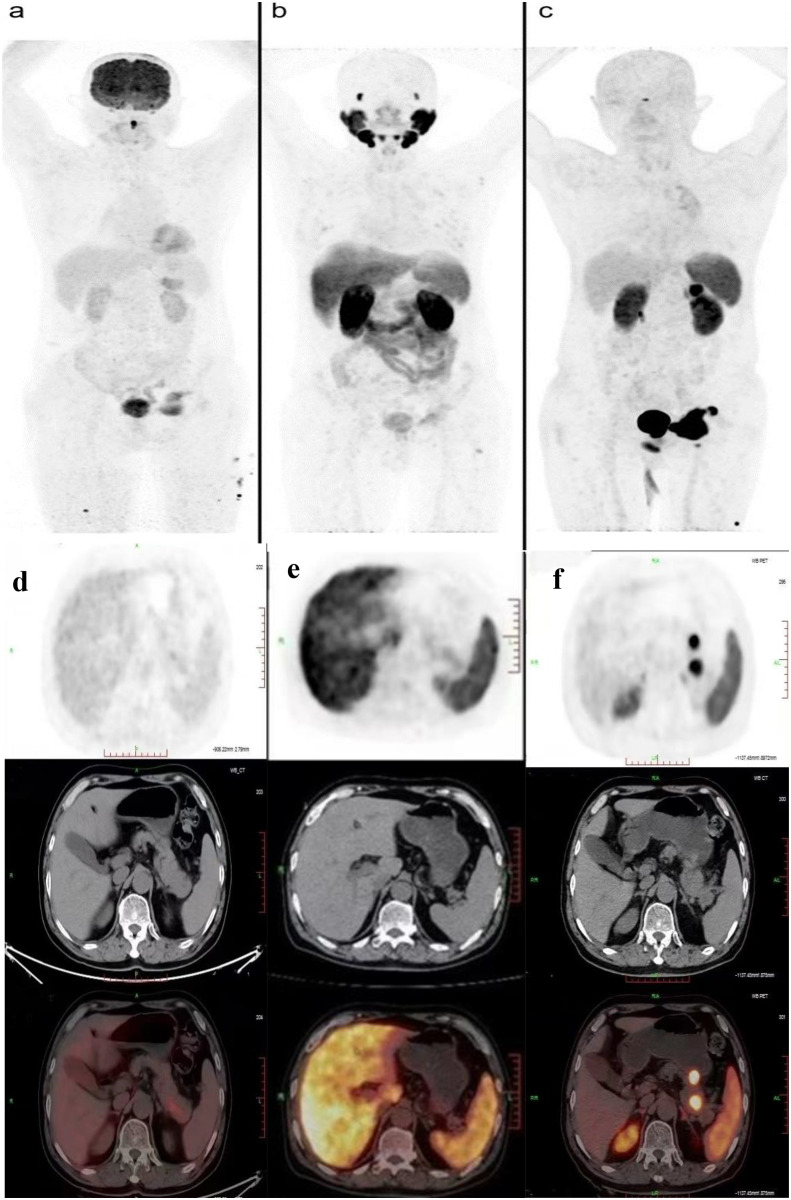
PET/CT showed comparison of the new lesions using 18F-FDG (A, D), 18F-PSMA (B, E), and 18F-JR11 (C, F) tracers.
